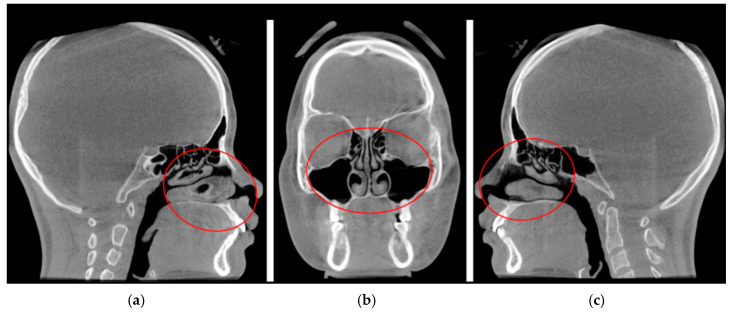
Pre-treatment CBCT assessment of paranasal sinus patency. Representative images show clear paranasal sinuses and patent sinus ostia (red circles). The absence of mucosal thickening ensures an accurate baseline for subsequent volumetric quantification in the (a) right sagittal, (b) coronal, and (c) left sagittal planes.
